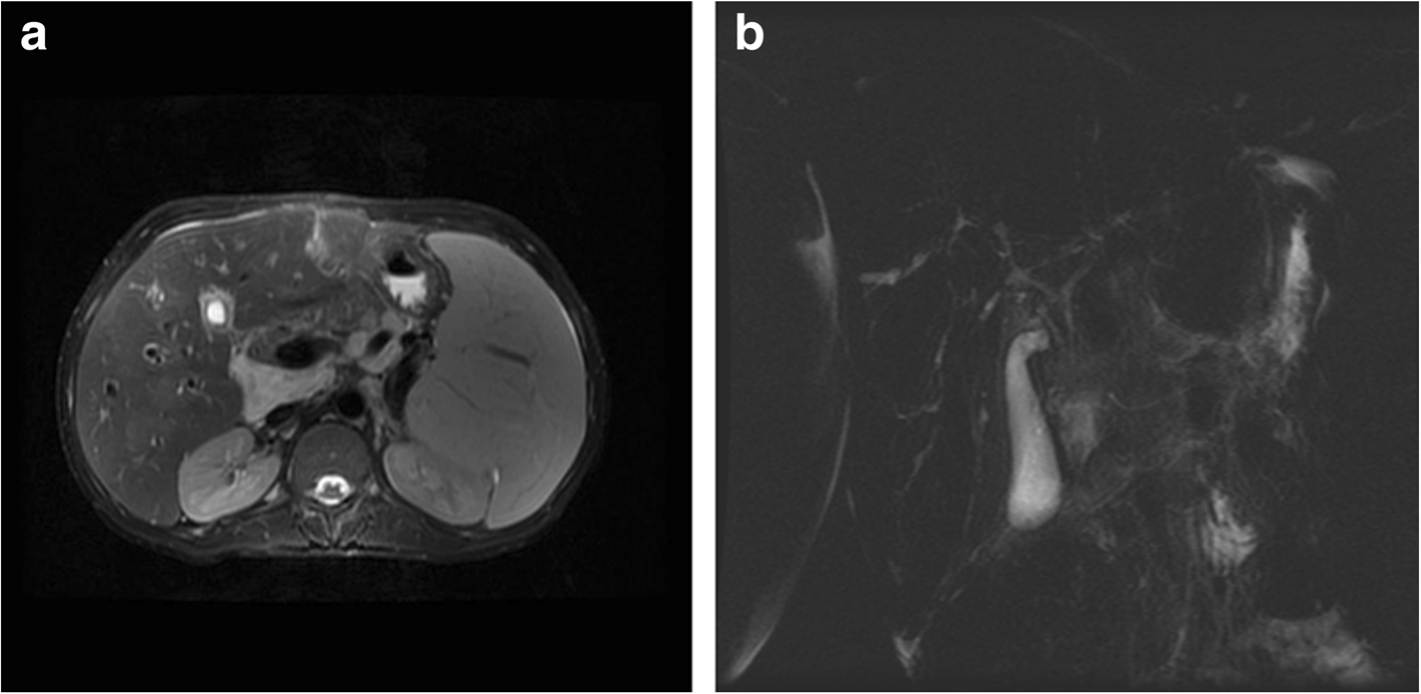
Magnetic resonance showing bile duct irregularities (a); T2-weighted image, depicting hepatic hilum tissue with contrast enhancement (b).
